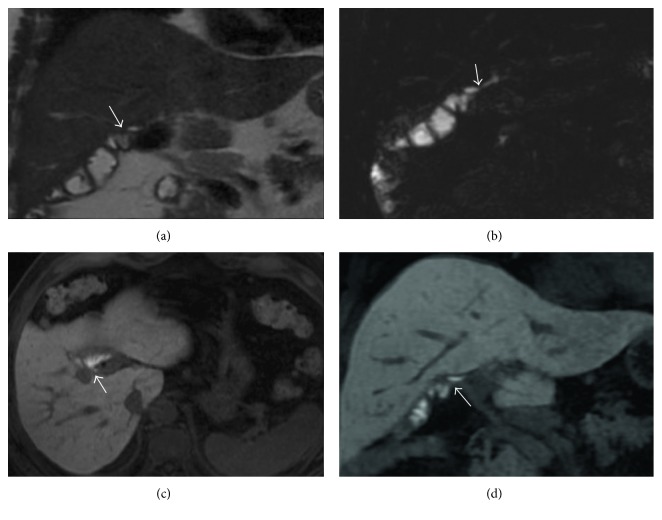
A 60-year-old patient with a biliary-enteric anastomoses. Coronal T2-weighted image (a) and 3D MRCP (b) clearly provide morphological representation of biliary-enteric anastomoses (white arrows). Contrast-enhanced MRC images show opacification of anastomoses (white arrows in (c) and (d)), adding functional information to morphology.
